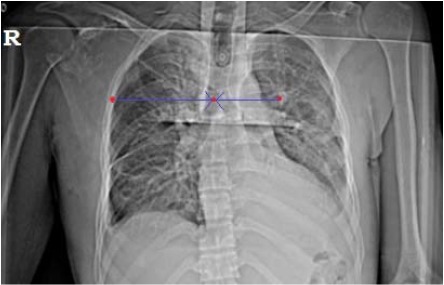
Fig. 2: CT with 3D reconstruction images showing scapulothoracic dissociation with comminuted fracture of scapula and acromioclavicular joint disruption and associated avulsion fractures of the spinous processes of vertebrae (D3-D5) F2:
